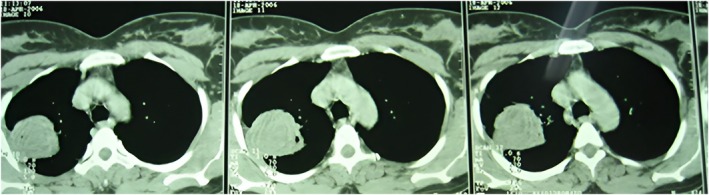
Axial non‐enhanced CT (pulmonary window): A large pulmonary opacity at the right lower lobes which is associated with subtle surrounding pulmonary inflammatory change on the form of surrounding ground glass opacity. Coronal CT image showed pulmonary lesion and central fluid attenuation. The fine needle aspiration needle tip is noted within the presumed wall of the cavitary lesion (not centre).
